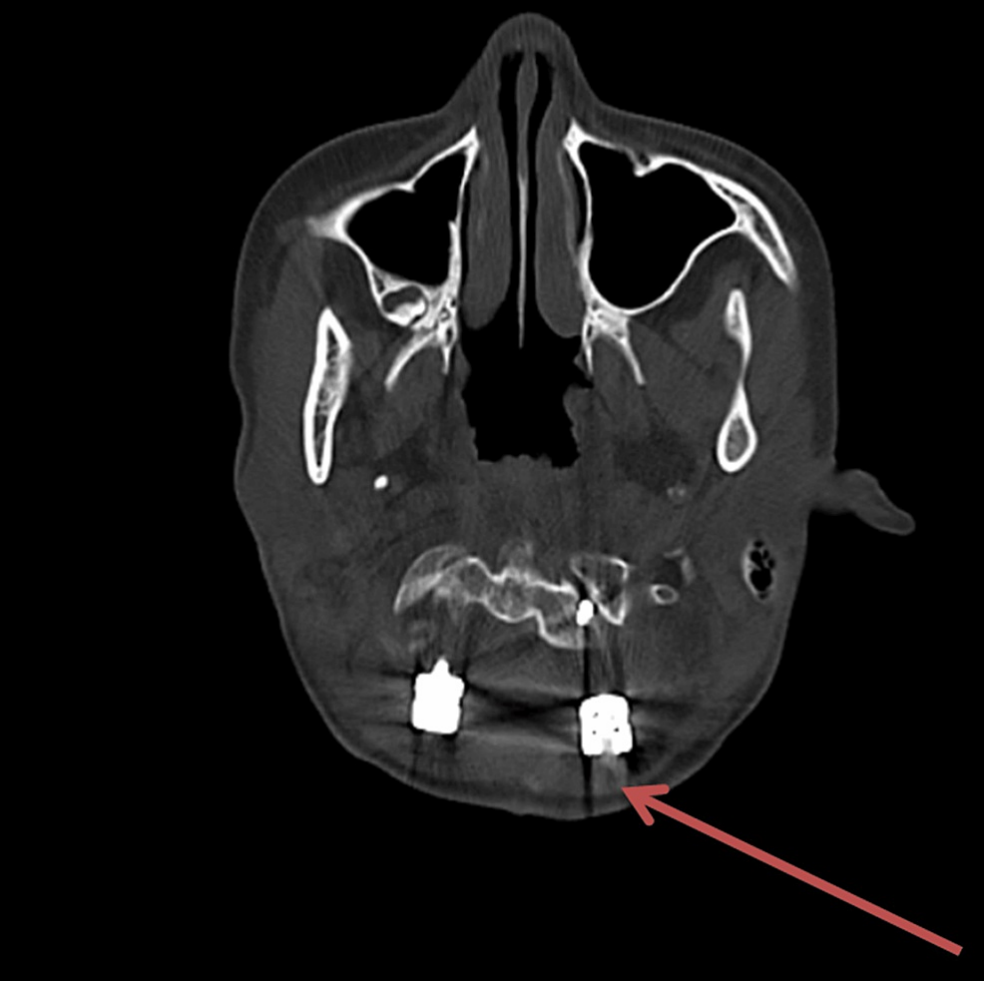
Brain CT scan showing the atlantooccipital assimilation with the C2-C3 fusion and basilar invagination compressing the cervico-medullary junction
